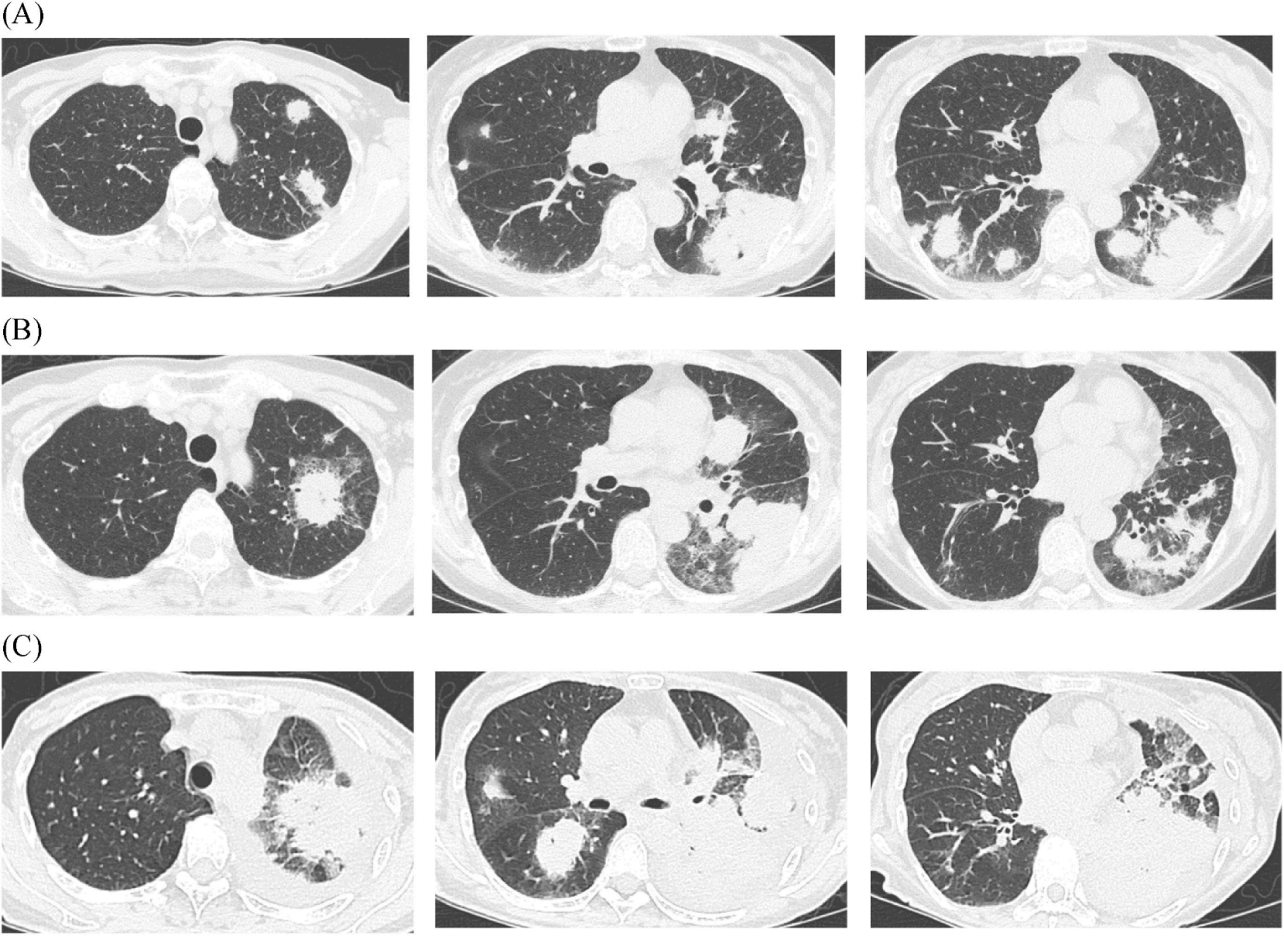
Chest CT images of the patient. (A) Image at first visit to our hospital showed multiple tumors and nodules in the bilateral lung fields. (B) Image at 4 months after first visit showed shrinking of tumors in the bilateral lower lobes and an enlarged nodule in the left lung. (C) Image at 7 months after first visit showed nodules were enlarged mainly in the left lung and a tumorous shadow emerged in the right lower lobe.
